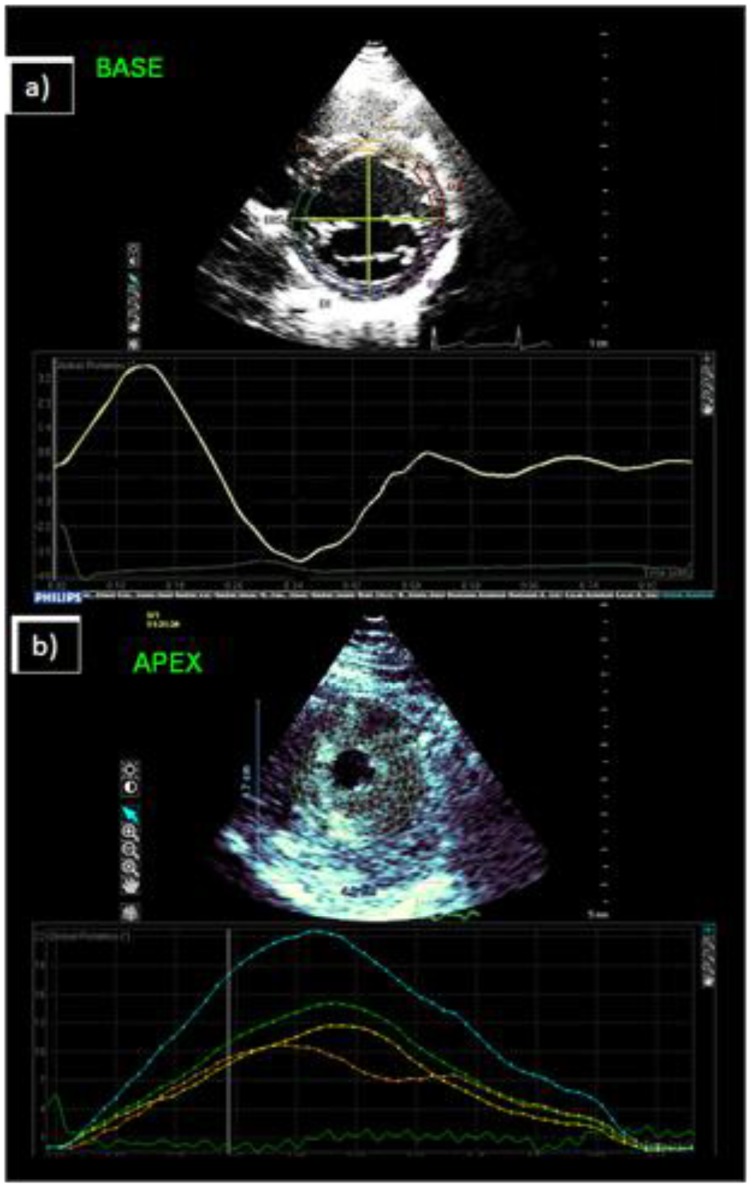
Speckle tracking echocardiography examples. A. Qlab 7.0, this is an example of speckle tracking at the LV base level. A single layer of tracking points is placed on either the endocardium or epicardium ∼60° away from each other. B. Qlab 8.1, an example of the LV short axis at the apex level. A mesh tracks all layers of the myocardium simultaneously.
